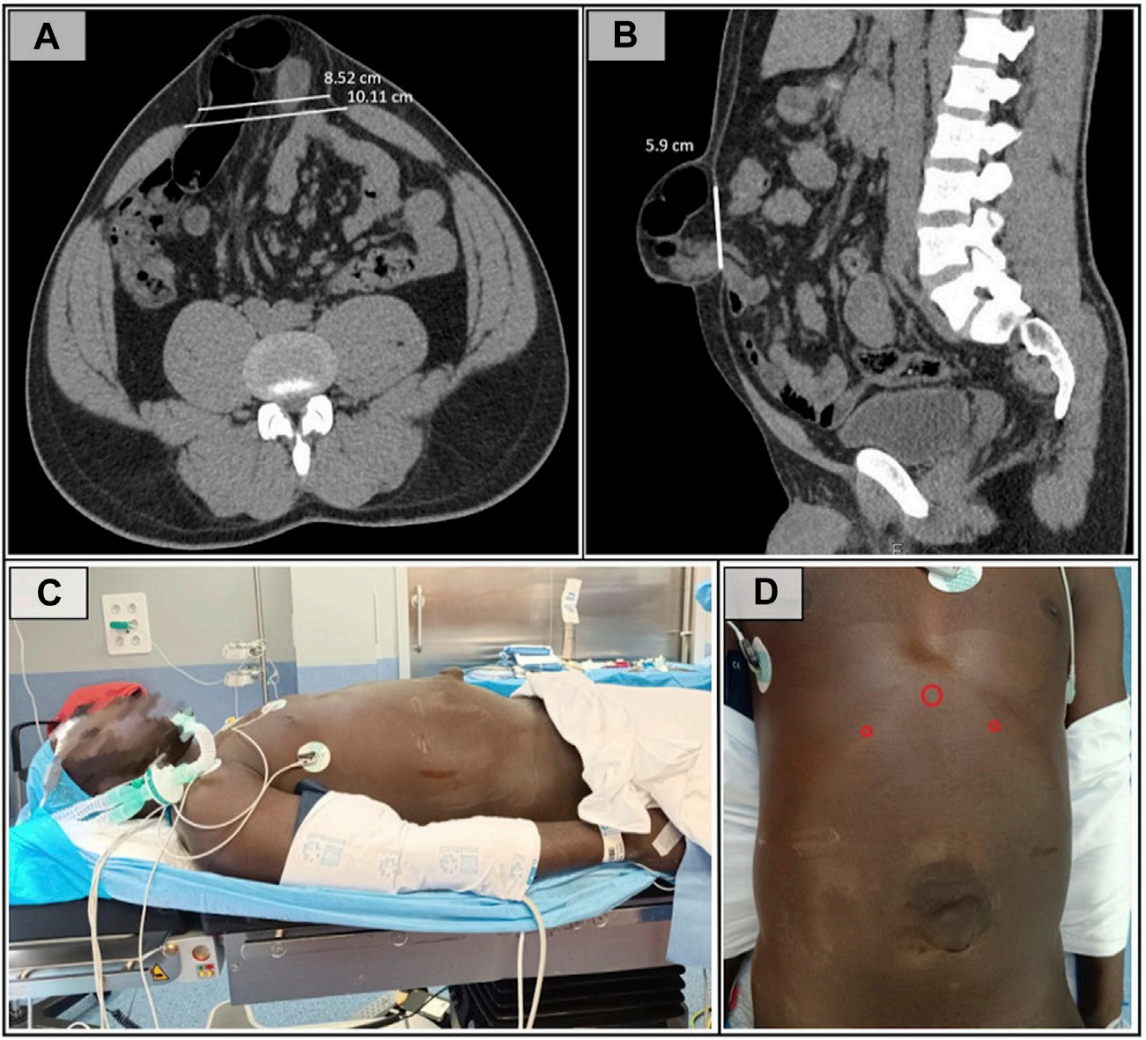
(A) Preoperative CT cross-sectional image showing a defect with a transverse diameter of 8.5 cm. (B) Saggital CT section demonstrating the 6 cm lenght of the defect. (C) Patient in the supine position with arms adducted. (D) Trocar placement showing a 12 mm subxiphoid port and 5 mm trocars in both hypocondria.
